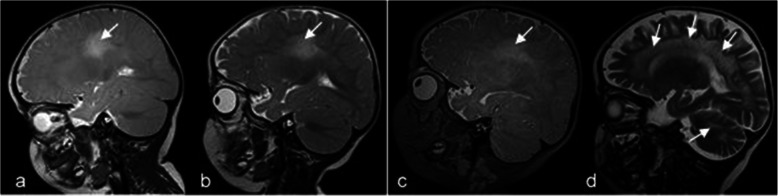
Pattern A2: MR images of a late-infantile patient in the disease course. Patient 28 (onset 9 months). At 11.5 months, the central white matter does, while cerebellar white matter does not show abnormalities (a). One and 1 ½ months later, parieto-occipital white matter is increasingly involved, the cerebellar white matter still not affected (b,c). Six months later, frontal, central and parieto-occipital white matter as well as cerebellum are affected (d).
